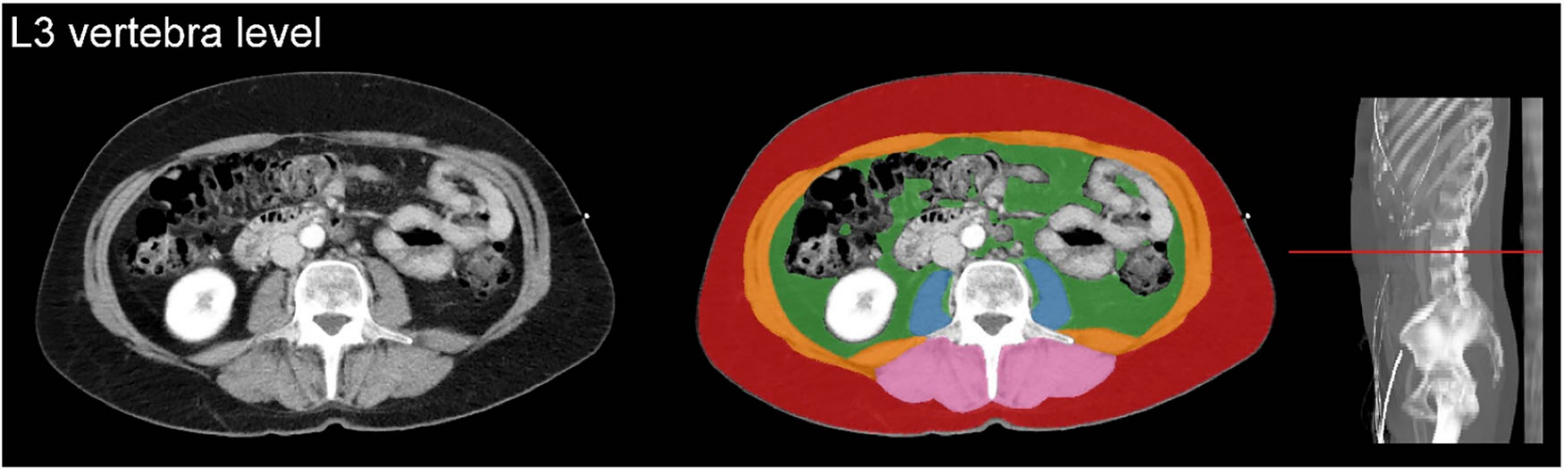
Artificial intelligence-driven segmentation of body composition on axial computed tomography images. Visceral fat (green), subcutaneous fat (red), psoas muscles (blue), abdominal wall muscles (orange), paraspinal muscles (pink)
